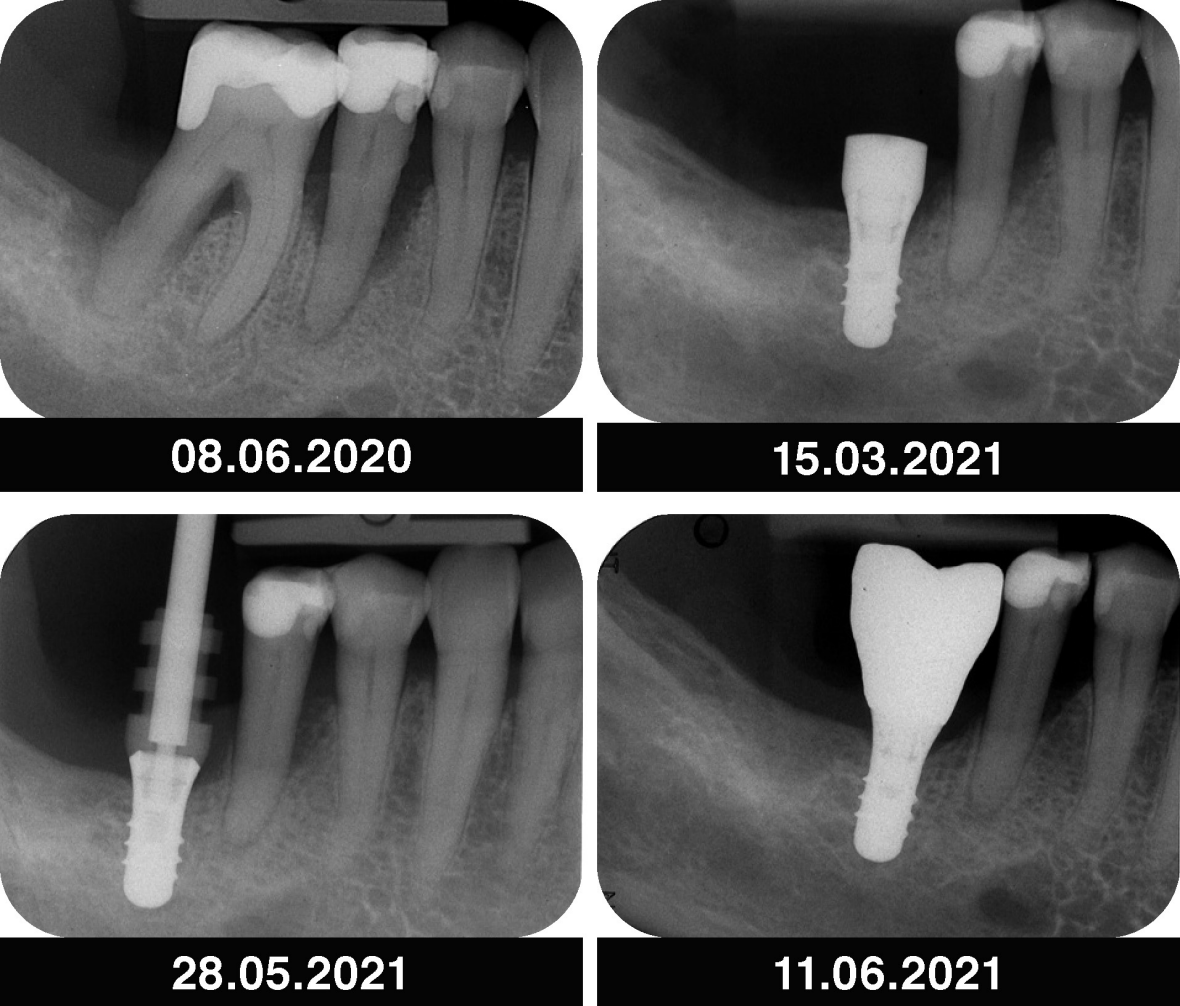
Radiographic images depicting the baseline situation and after implant placement and prosthetic restoration
